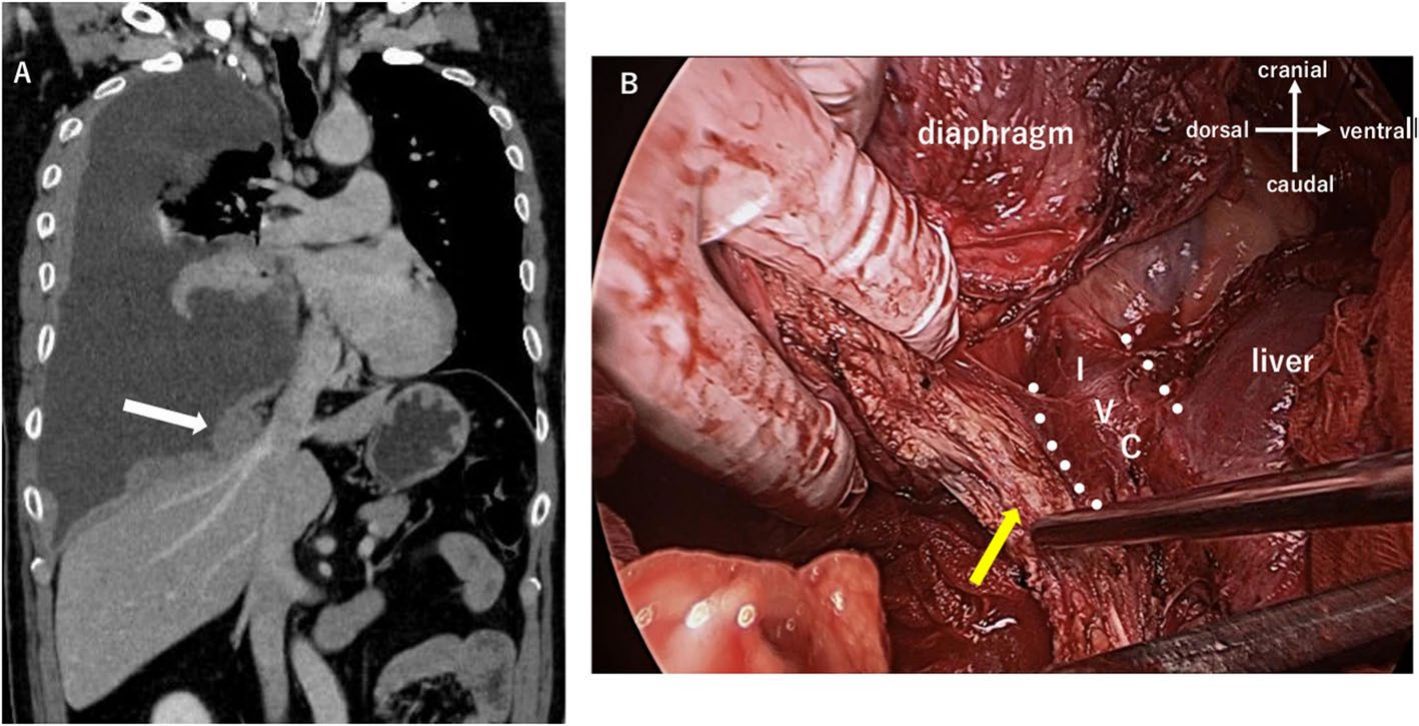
A Findings of contrast-enhanced computed-tomography (CT) scan. The tumor was very close to the inferior vena cava (IVC) and hepatic veins (white arrow). B Intraoperative photo viewed from the abdominal side. There was a large amount of tissue around the IVC, where the dissection was most difficult (yellow arrow)
